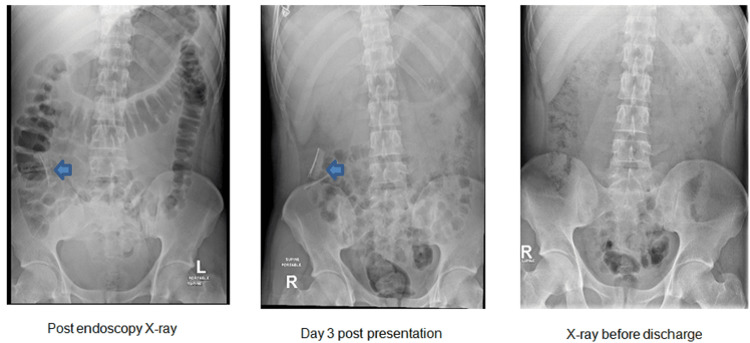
A sequence of X-ray images indicating different times during the hospital stay
